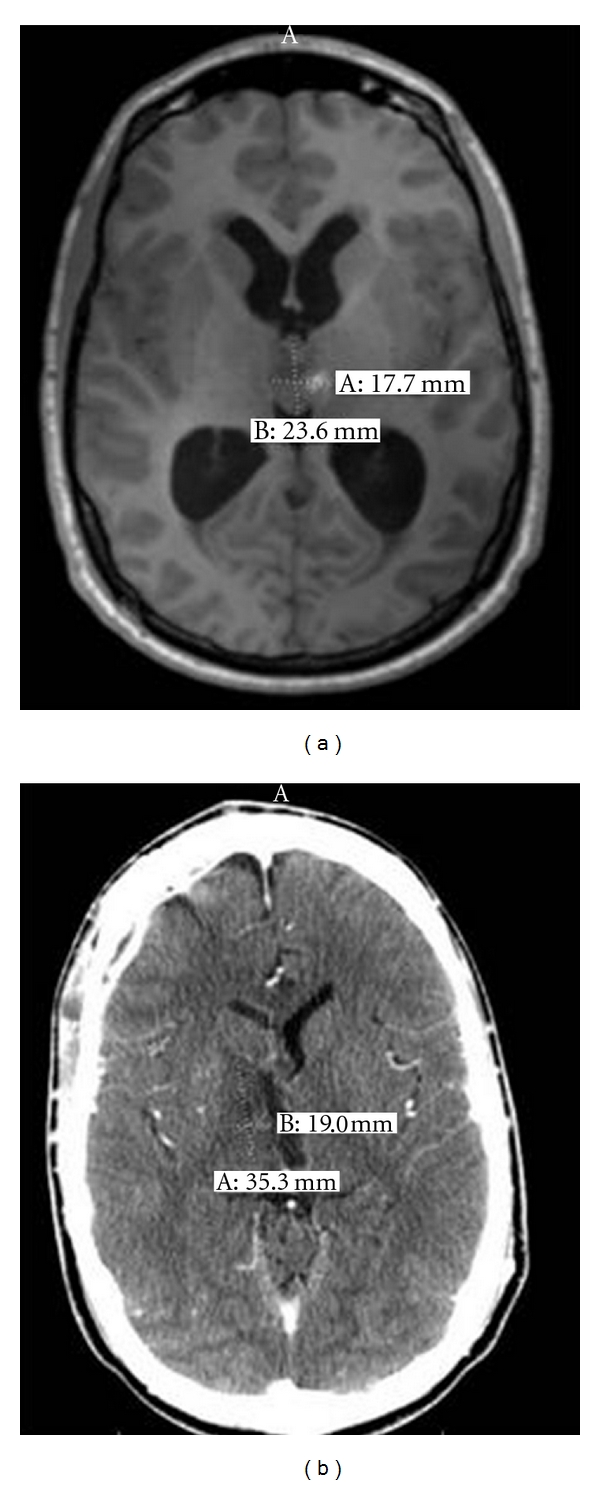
Participant no. 4 with a right thalamic lesion. The MRI image in (a) depicts a calcified third ventricular mass occluding the third ventricle which was subsequently resected. Lateral ventricular dilation is evident. The CT image in (b) depicts resolution of ventricular dilatation subsequent to tumour resection and reopening of the cerebrospinal fluid cisterns. However, (b) shows a right posterior thalamic ischemic infarct appearing two weeks after resection of the third ventricular tumour.
